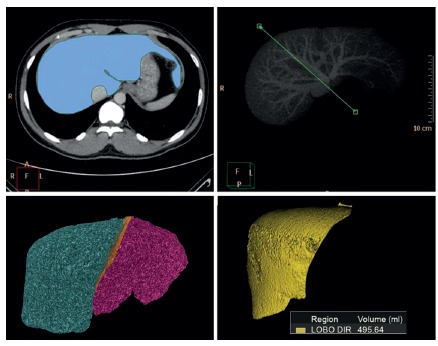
Images from pre operative CT hepatic graft volumetry of living donor: A) delimitation of whole hepatic area for CT volumetry; B) green line demarking medium hepatic vein, this line splits right and left hepatic lobes; C) right and left hepatic lobes; D) virtual right hepatectomy with estimated volume
